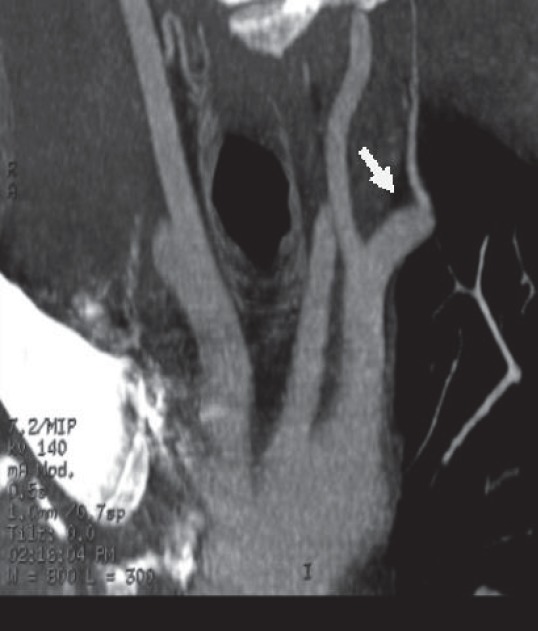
Angio-CT left ICA dissection
